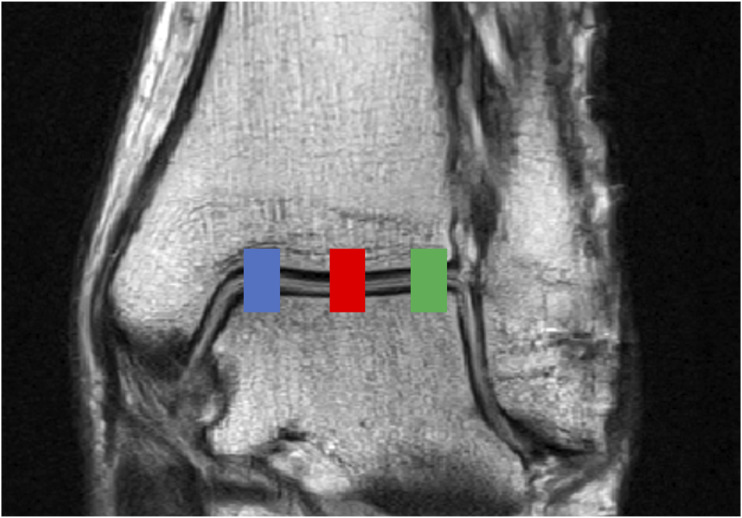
Coronal T2-weighted image of ankle joint from a 43-year-old male for demonstration purposes. Each of the studied three sections is indicated by their respective color: blue = medial section, red = central section, and green = lateral section.
